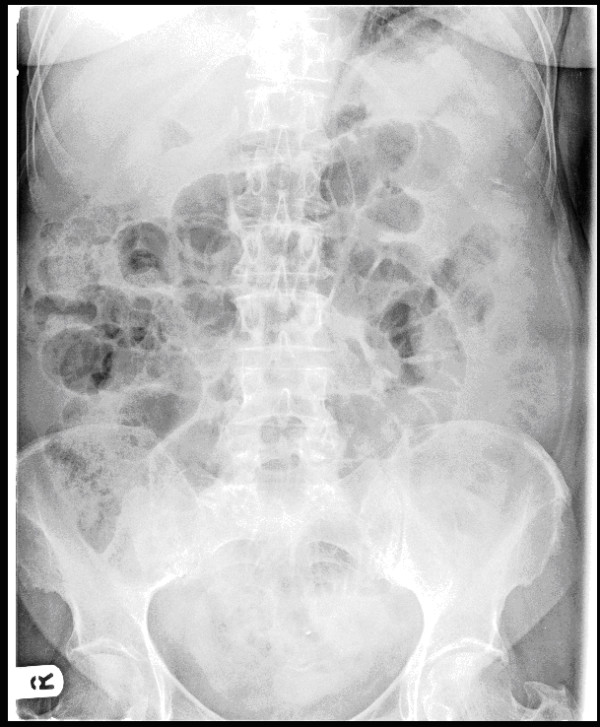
Abdominal X-Ray on the day of A&E admission showing small bowel dilation.
